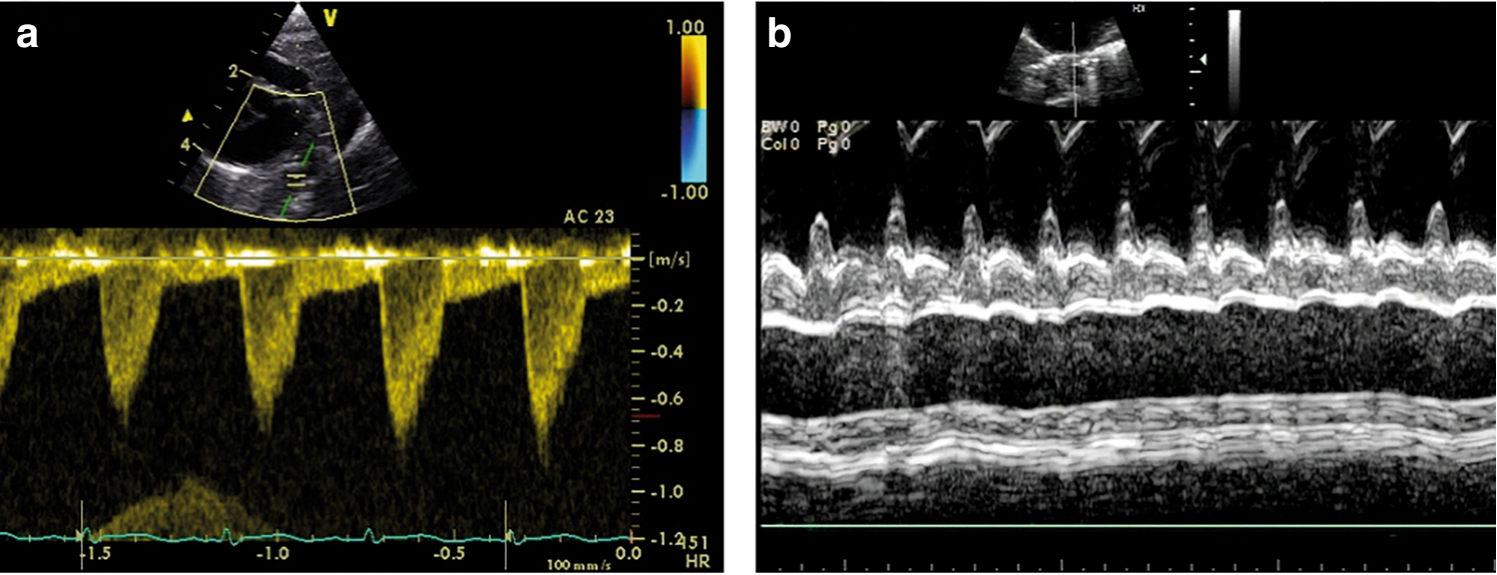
Assessment of descending aorta (DAo) flow. The velocity–time integral (VTI) of DAo flow can be assessed both from a high parasternal or low subcostal sagittal view using pulse-wave Doppler with a minimum angle of insonation (a). In the presence of any retrograde diastolic aortic blood flow, the DAo flow should be corrected by deducting the retrograde VTI from the antegrade VTI. The diameter of the DAo is measured in the short axis parasternal axis view, as close as possible to the plane of the aortic valve (b)
